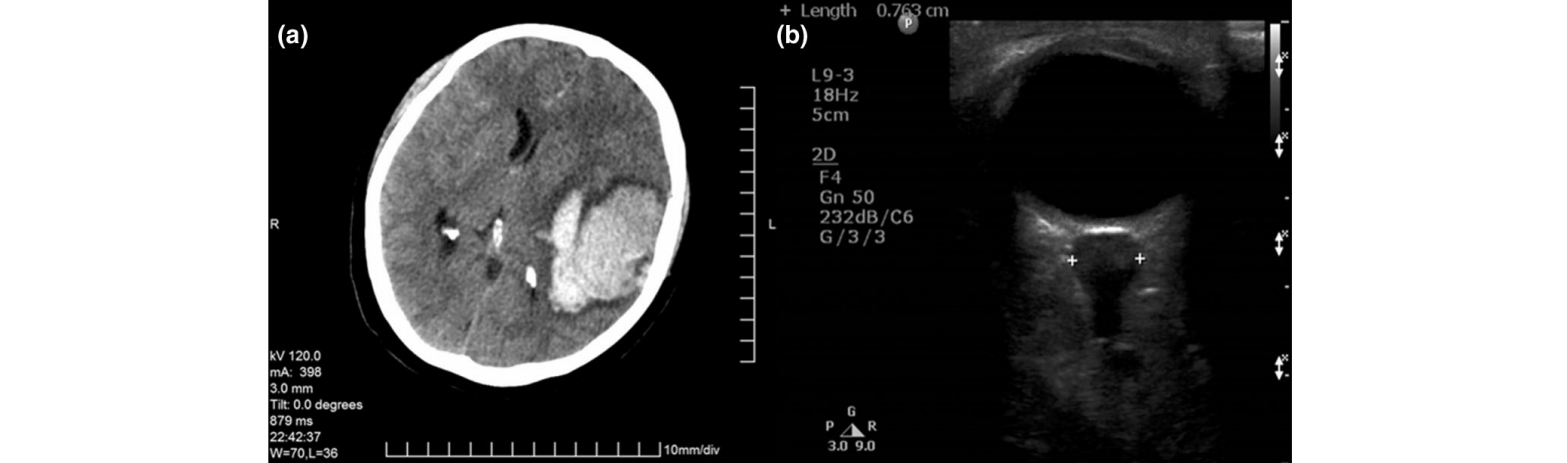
Imaging findings of a brain-injured adult. (a) Brain computed tomography (CT) scan of a patient showing a midline shift of more than 5 mm and a nonevacuated lesion of more than 25 ml. (b) Transorbital sonography of the same patient documenting increased optic nerve sheath diameter.
